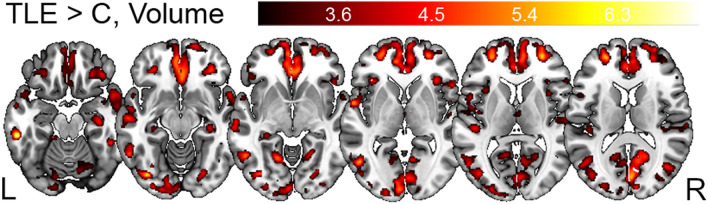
Grey matter volume differences between left TLE patients and healthy controls. Statistical parametric maps (SPM) of grey matter volume differences between patients with left-lateralized TLE and healthy controls. SPMs displayed on axial T1-weighted image in standard MNI space. For visualization purposes SPMs thresholded at *p* < 0.001, uncorrected for multiple comparisons. *TLE* temporal lobe epilepsy, *C* healthy controls, *L* left, *R* right hemisphere
